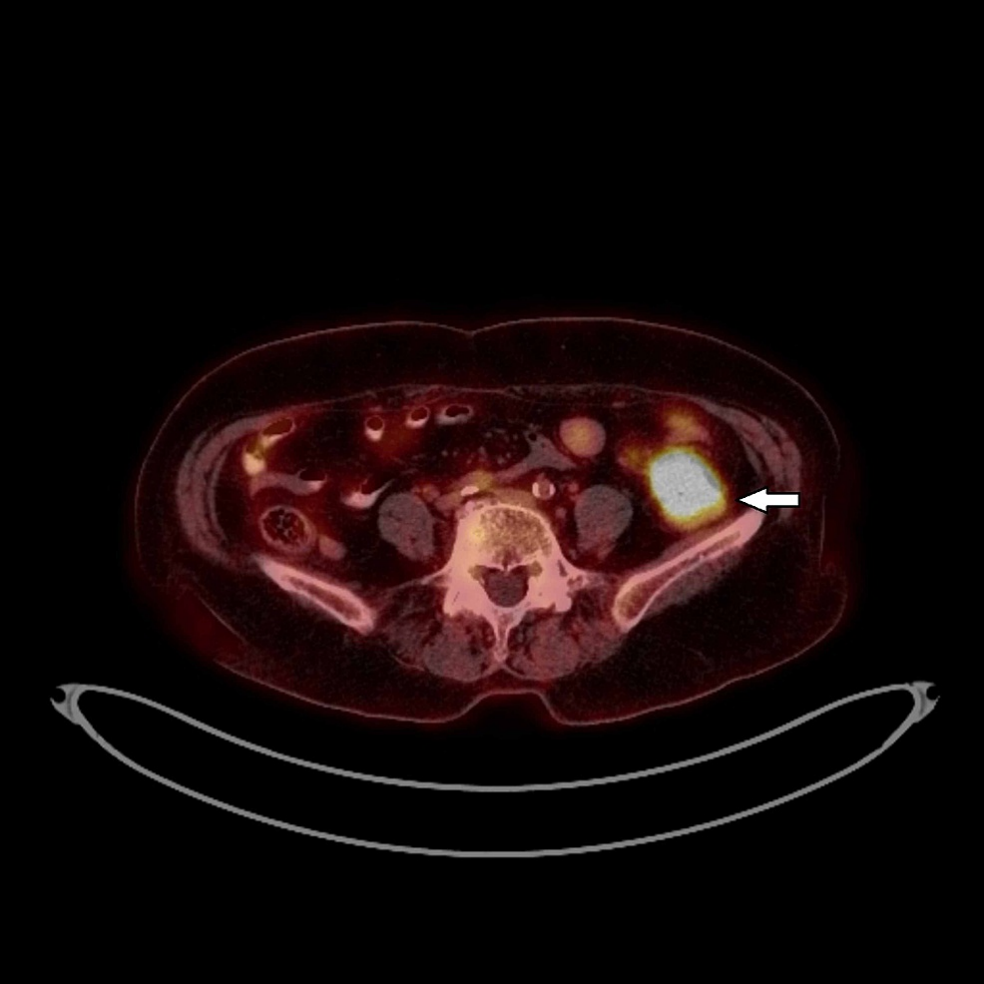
Pre-operative PET-CT showing the descending colon metastasis (arrow) from the squamous cell lung carcinoma. PET-CT: positron emission tomography-computed tomography.
